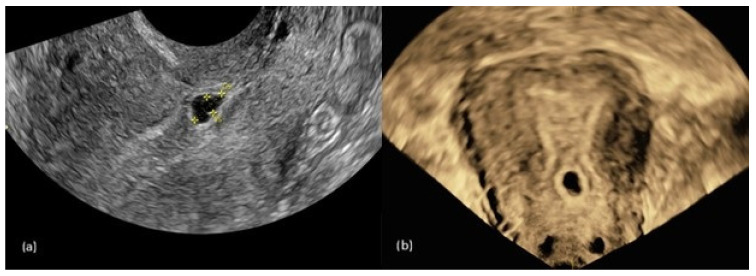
(a) Two-dimensional transvaginal ultrasound image and (b) three-dimensional transvaginal ultrasound image showing a gestational sac located in the anterior lower uterine segment at the site of the previous cesarean scar.
